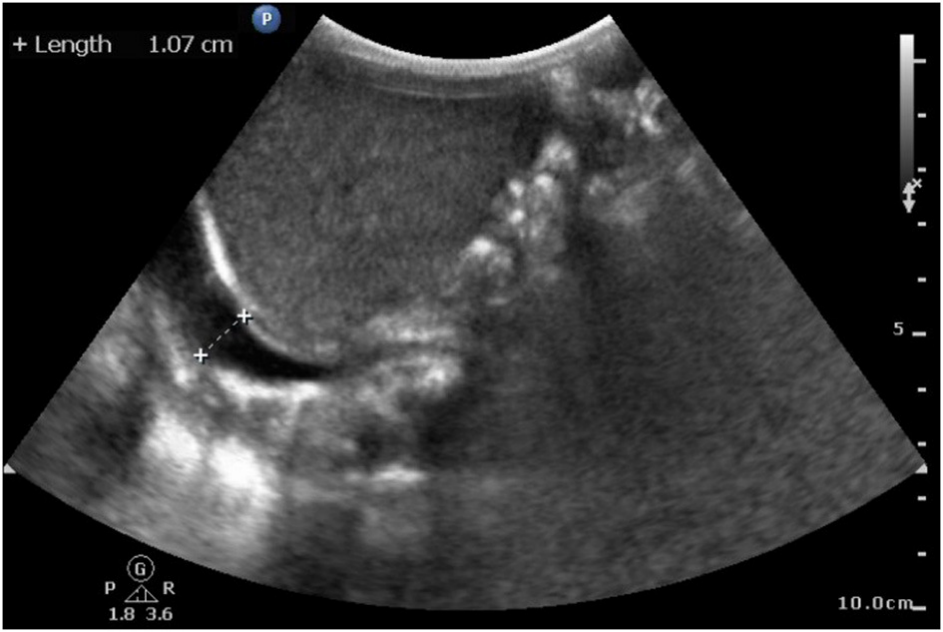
Sonography at 43 days of age. Hemothorax detected by chest sonography after first discharge without prophylaxis. Image shows the accumulation of clear fluid in the right pleural cavity under infra-hepatic view.
